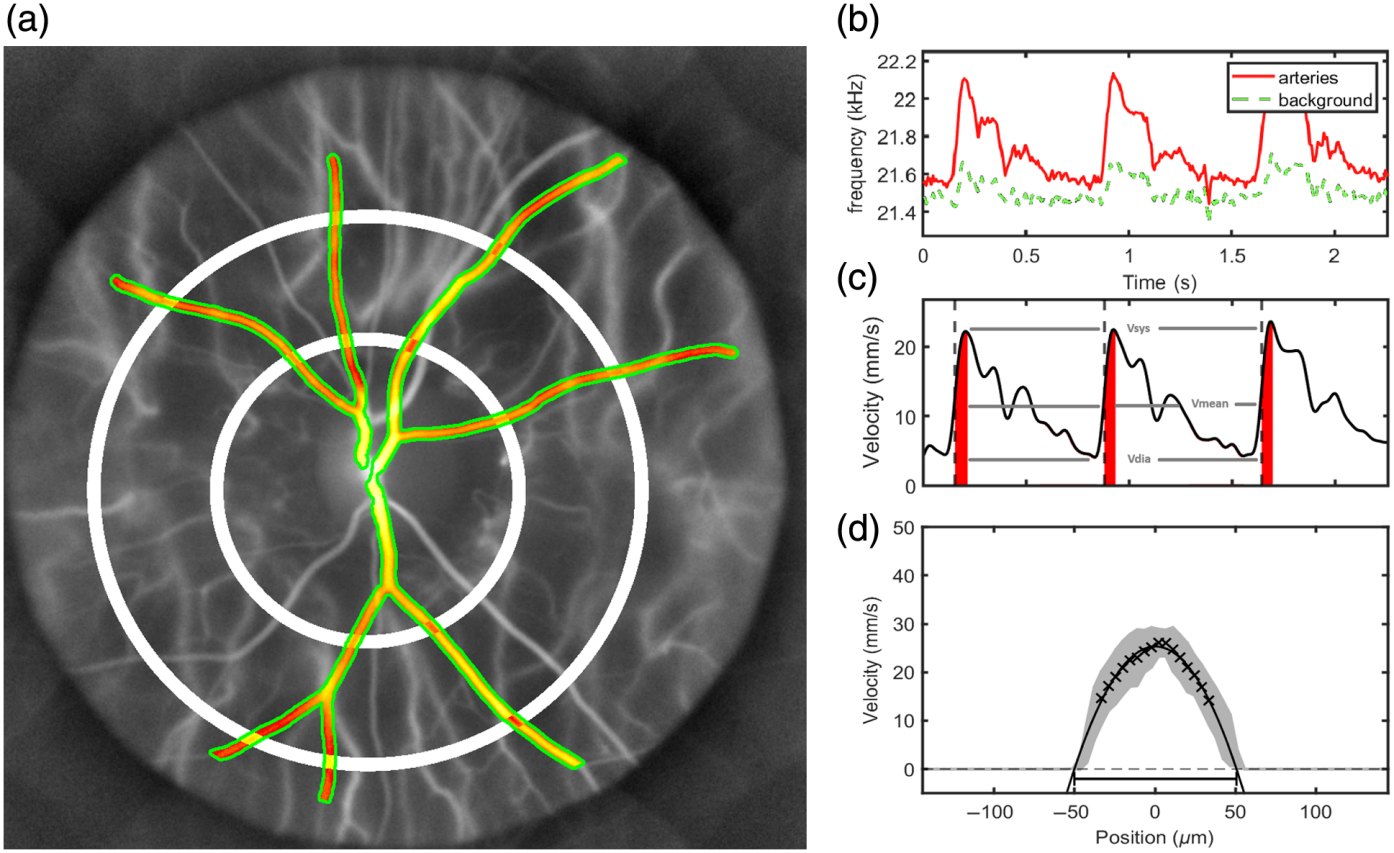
(a) Time-integrated hemodynamic velocity map showing segmented arterial regions (red), surrounding background tissue (green), and white annular regions used for spatial averaging. Spatially averaged Doppler frequency broadening measured in arterial regions (red) and background tissue (green) (b). Arterial waveform showing mean, systolic, and diastolic velocity, obtained by subtracting background from arterial frequency broadening, applying Eq. (1), and low-pass filtering over an integer number of cardiac cycles. The dark red area indicates the arterial stroke volume (c). Representative mean arterial velocity profile of a typical section used for diameter estimation (d).
